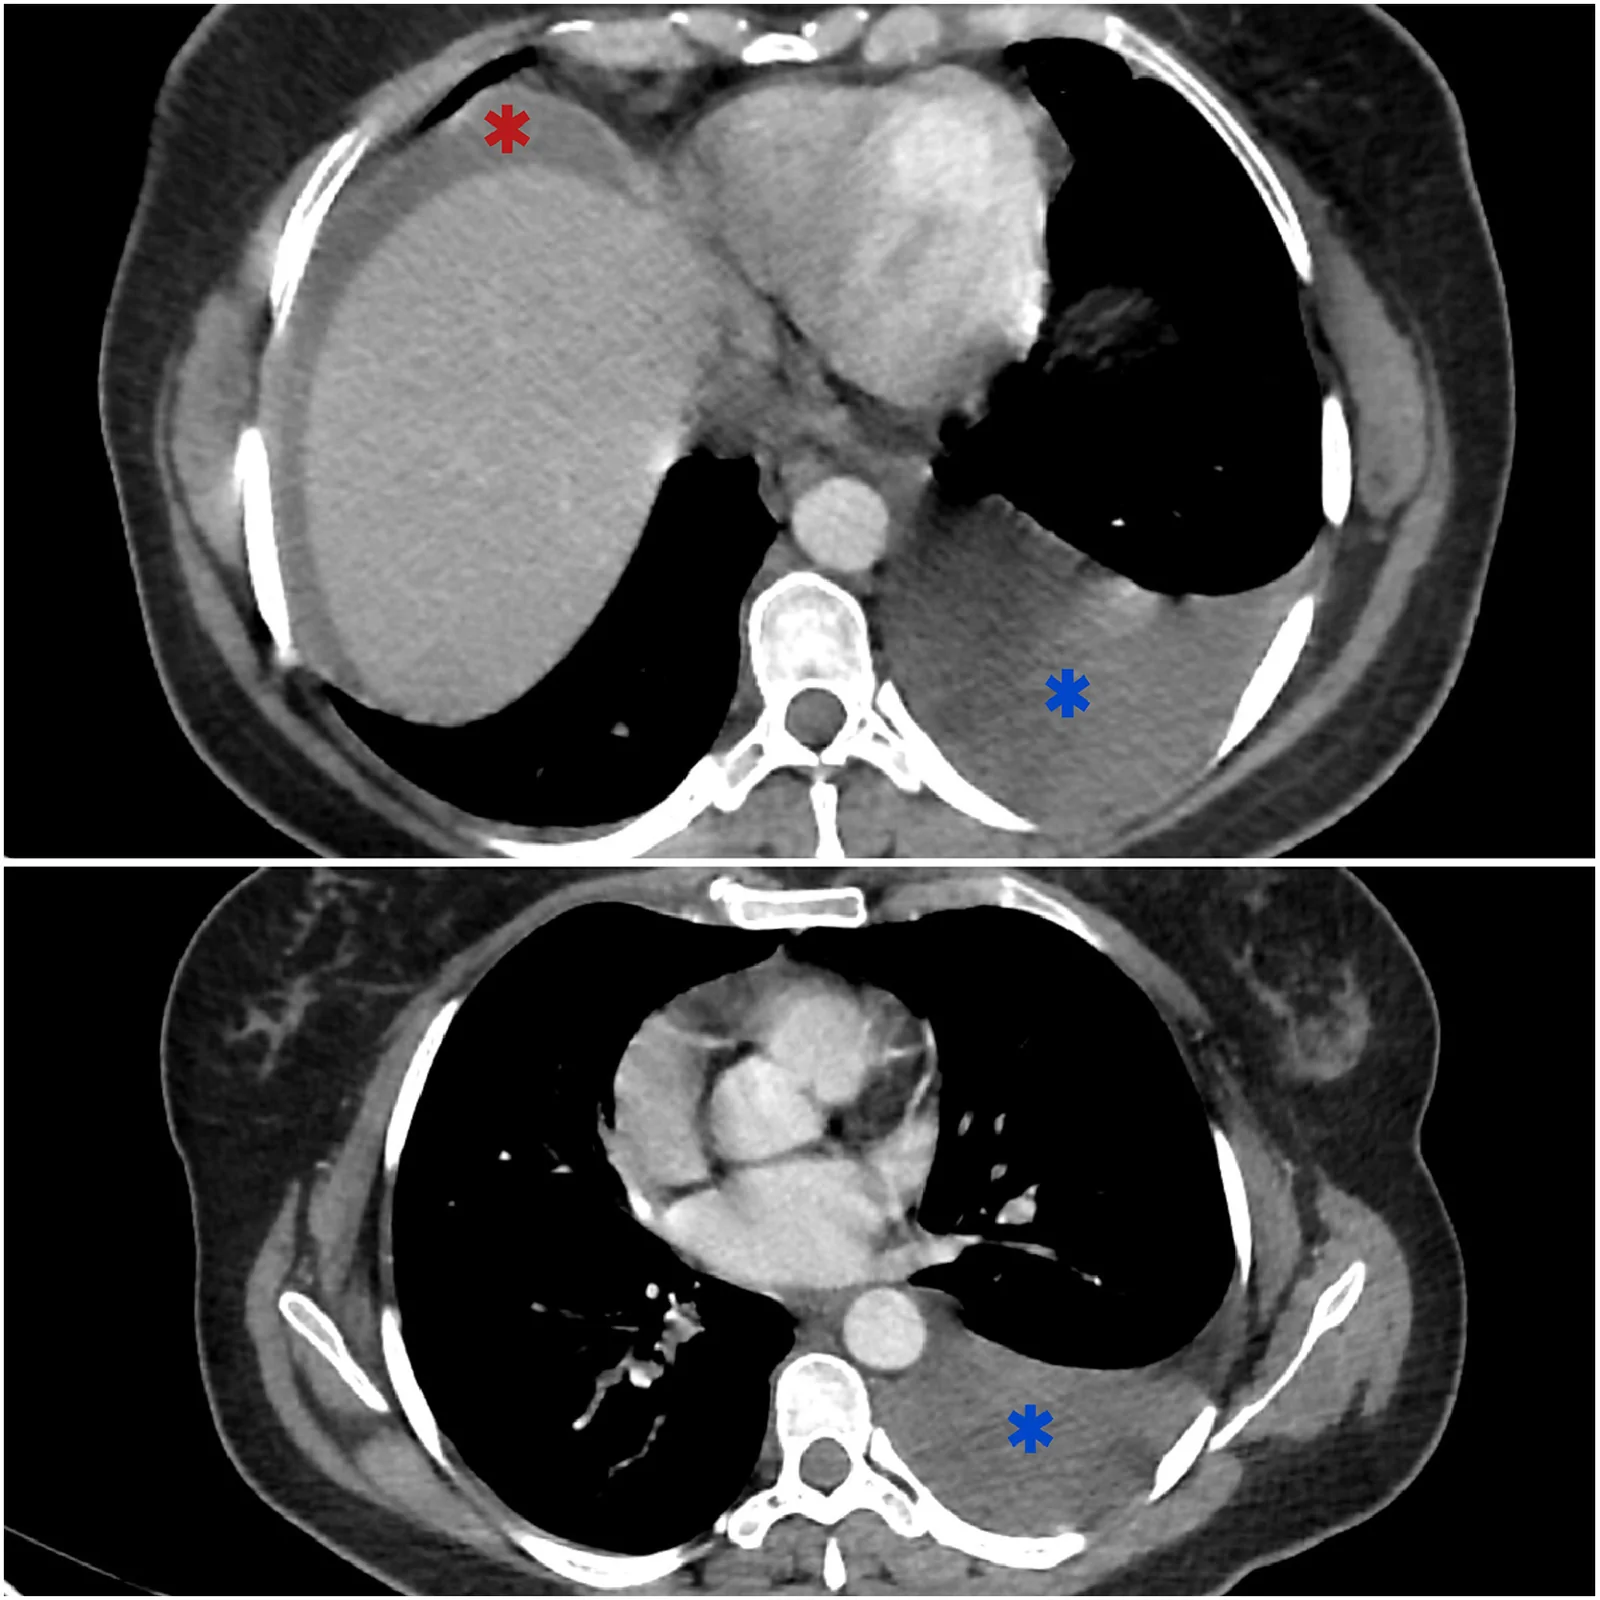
Axial contrast-enhanced CT images showing abundant intraperitoneal ascites (red asterisk) associated with left pleural effusion (bleu asterisk) in the setting of a giant right ovarian mass.
